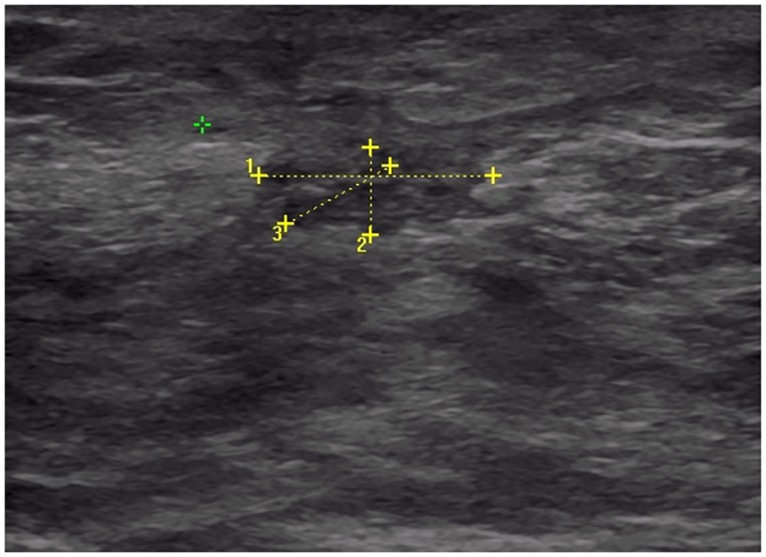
Ultrasound imaging of the wound. Image acquired in the transverse plane of the wound at week 19 after surgery. The segments 1 and 2 were measured at 13 mm and 4 mm, respectively.
